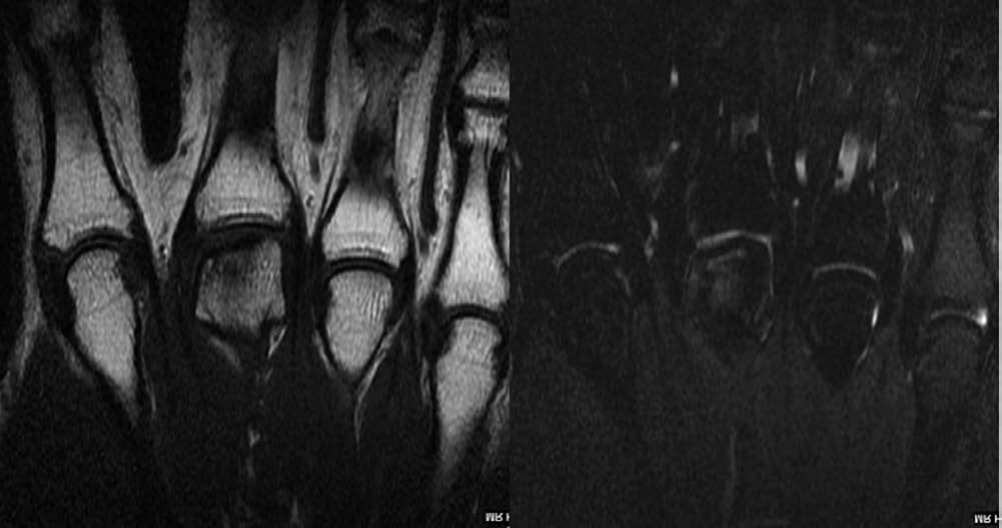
T1 weighted image showing bony union of the plug and T2 weighted image showing articular cartilage subsidence of the 3rd metacarpal head on magnetic resonance image at 12 weeks after the surgery.
